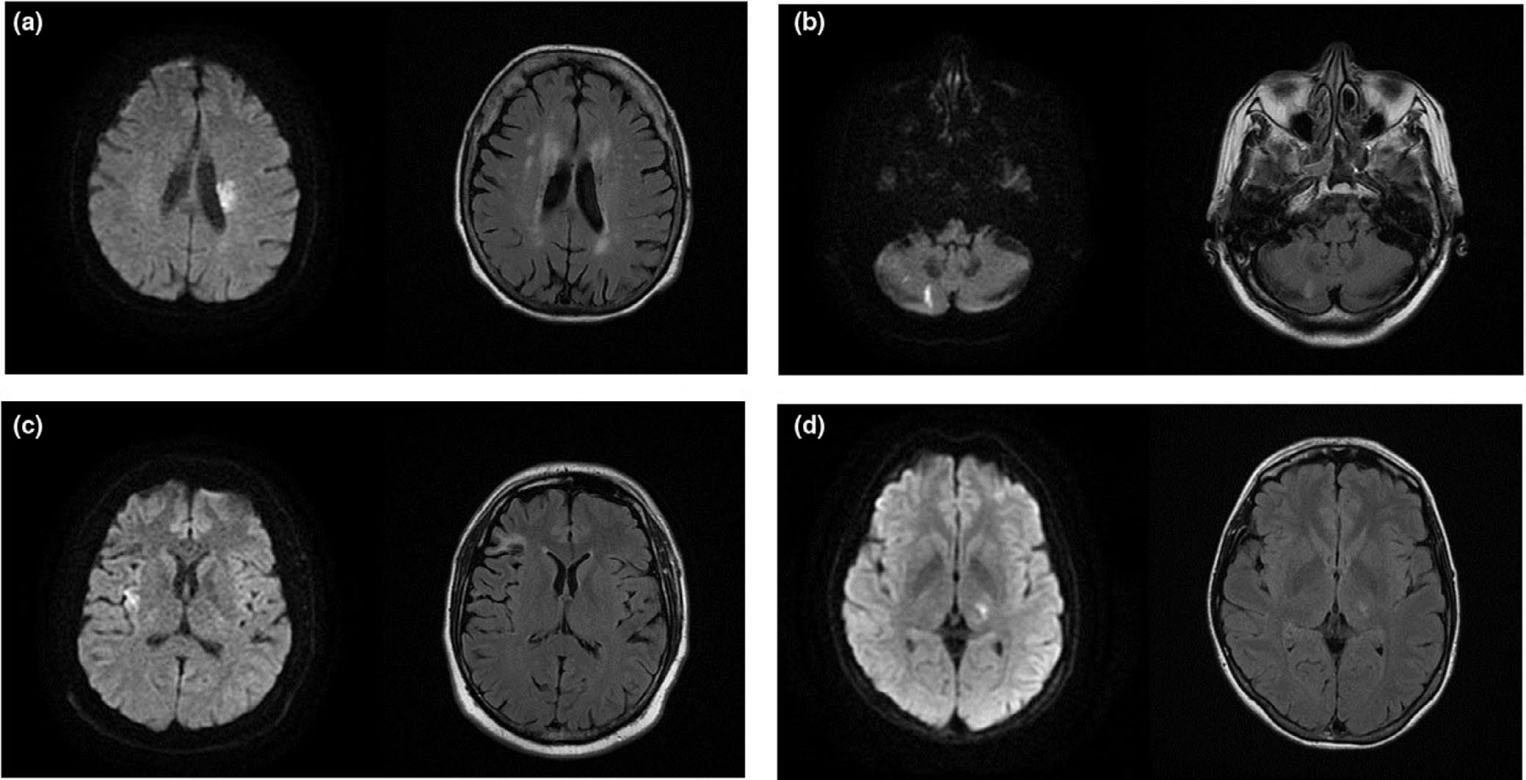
Diffusion‐weighted imaging (DWI) and fluid‐attenuated inversion recovery (FLAIR) mismatch in the selected cases of wake‐up stroke. (a) The clear DWI/FLAIR mismatch (left side) presented in case 2. (b) The acute ischemic lesions visualized 24 hr post‐thrombolysis on DWI (left side) and FLAIR in case 3. (c) The clear DWI/FLAIR mismatch (left side) presented in case 4. (d) The doubtful DWI/FLAIR mismatch (left side) presented in case 5
